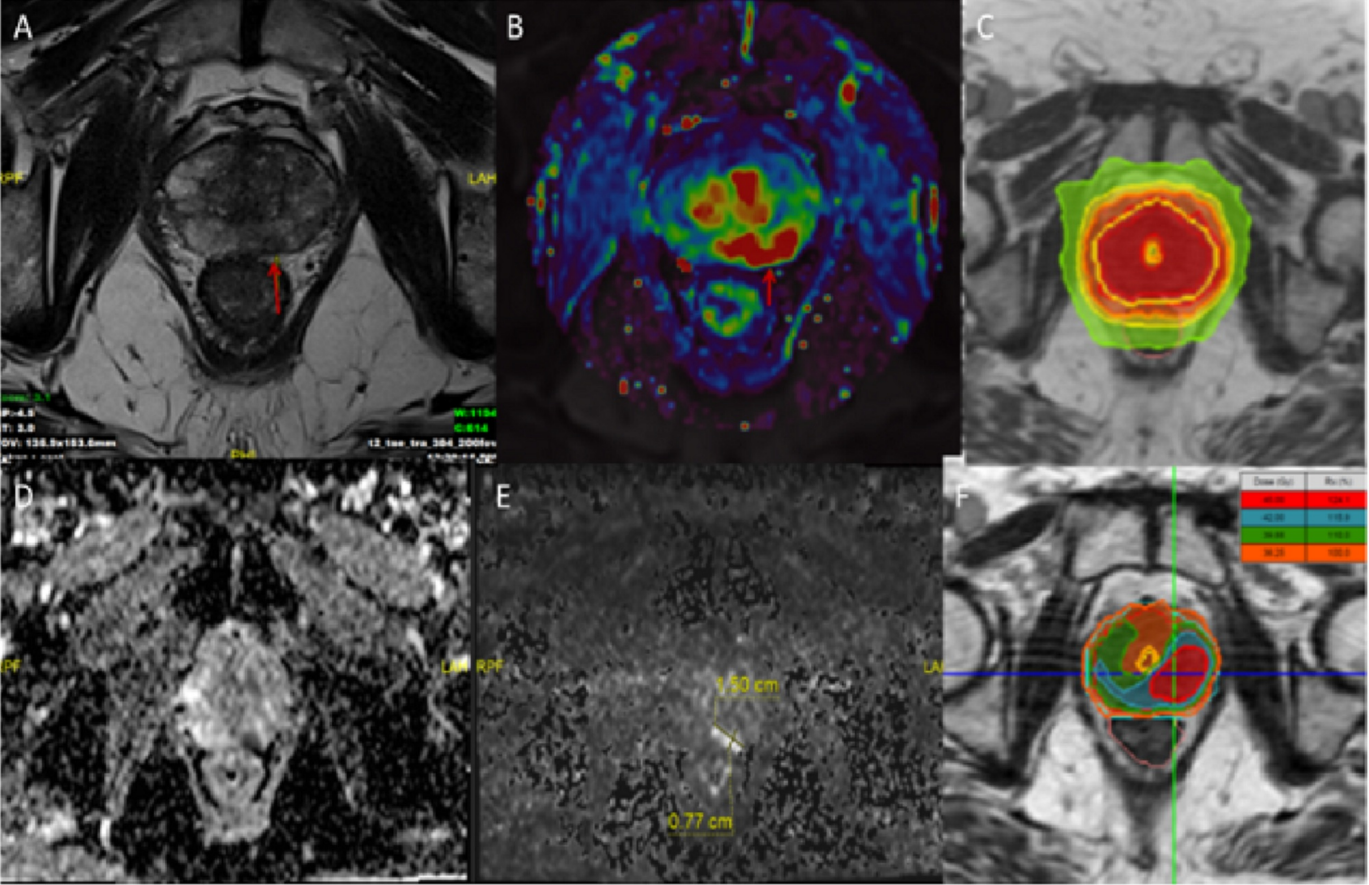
MRI scans and radiotherapy planning (A, B, D and E) Axial views of T2, K-trans, perfusion and diffusion MRI scans. (C) Homogeneous plan allowing homogeneous dose to whole prostate with urethral sparing. (F) Heterogeneous plan to escalate dose on resistant tumor segments.
